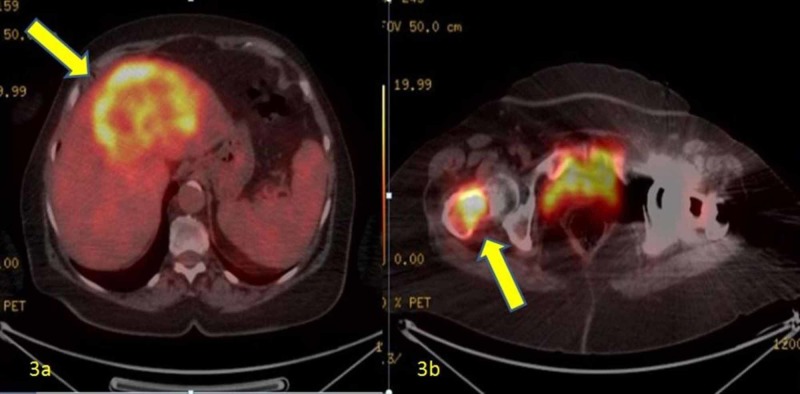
3a: Hypermetabolic malignant mass with axial dimensions of 12x10 cm in the liver in PET-CT; 3b: Metastatic mass in the proximal femur in PET-CT PET-CT: positron emission tomography-computed tomography
